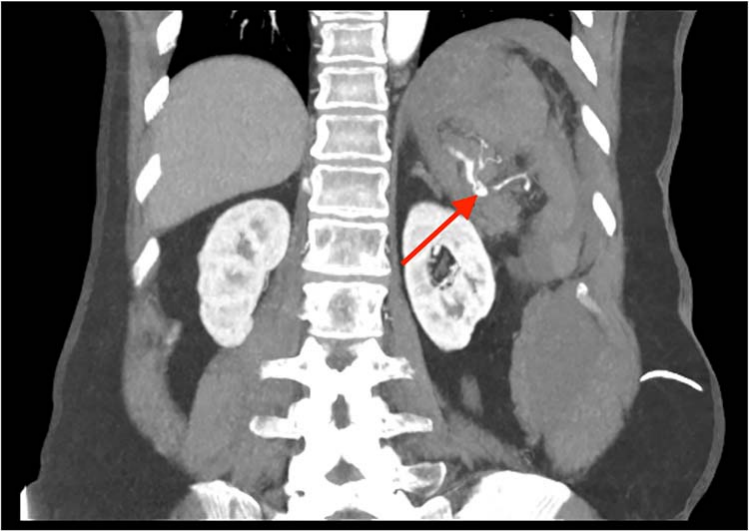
CT of the abdomen and pelvis, coronal plane, arterial phase. Contrast extravasation from the ruptured SAP.
